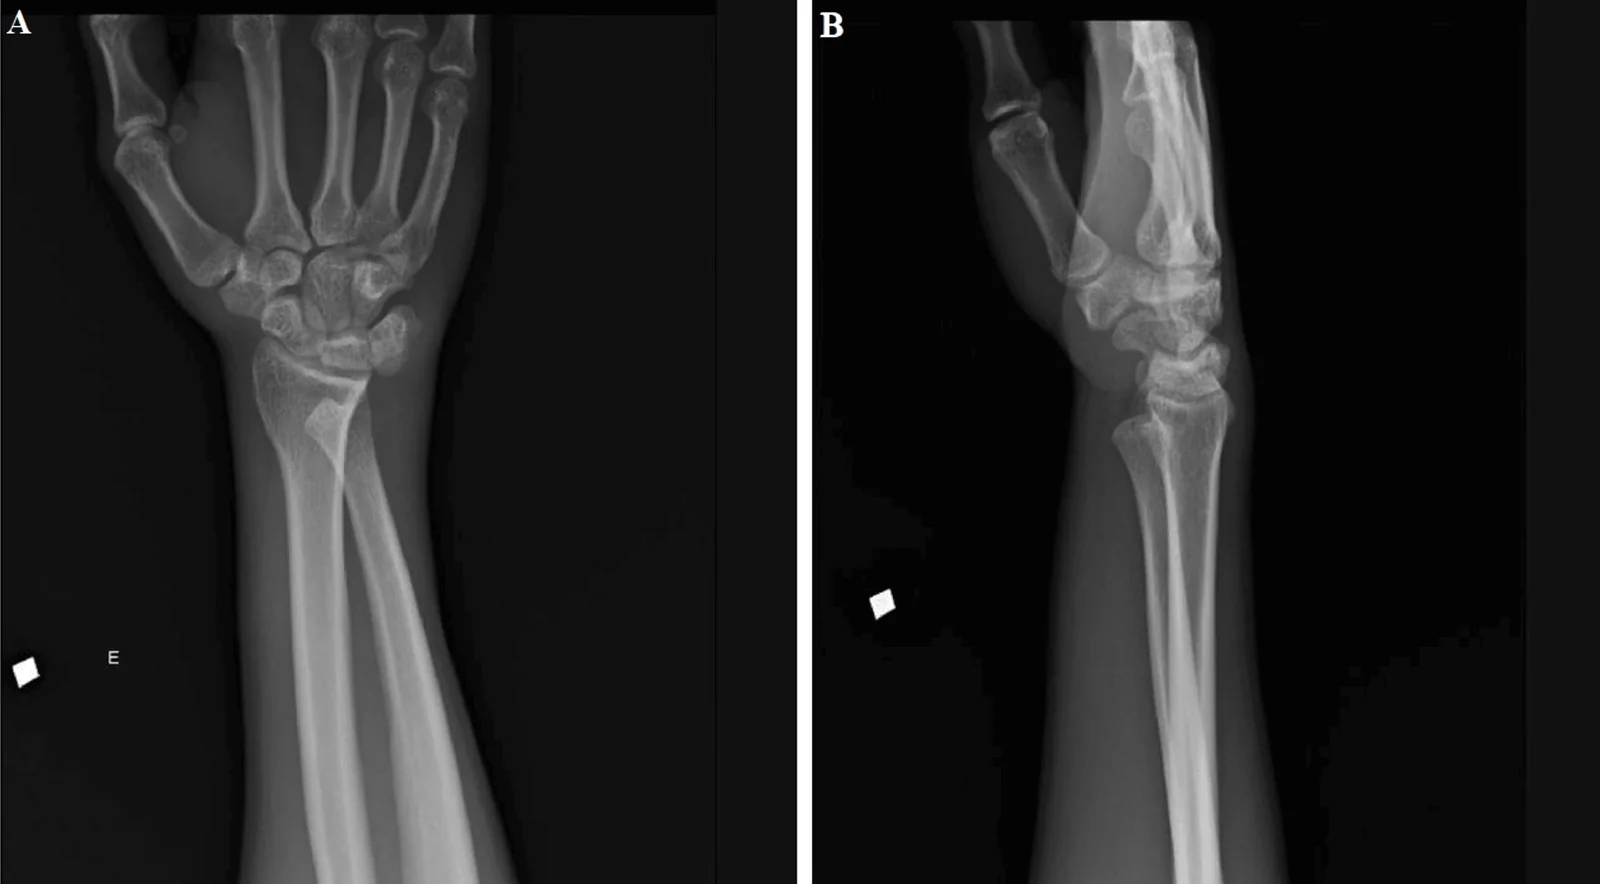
Initial radiographs of the left wrist. (A) Anteroposterior view. (B) Lateral view demonstrating isolated volar dislocation of the DRUJ without associated fracture. DRUJ: distal radioulnar joint
